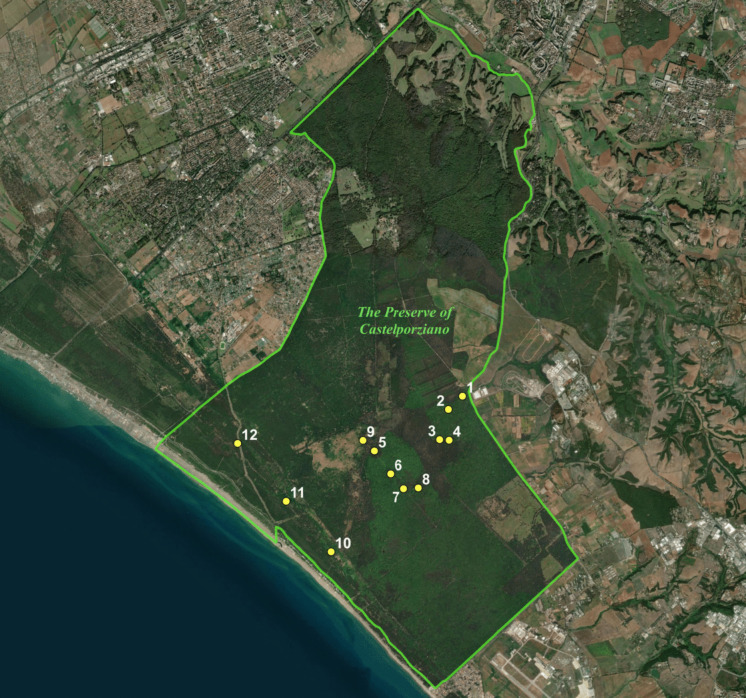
The Preserve of Castelporziano (Rome, Italy). Yellow dots represent the location of the experimental plot in the CT Detection experiment (2.1)
